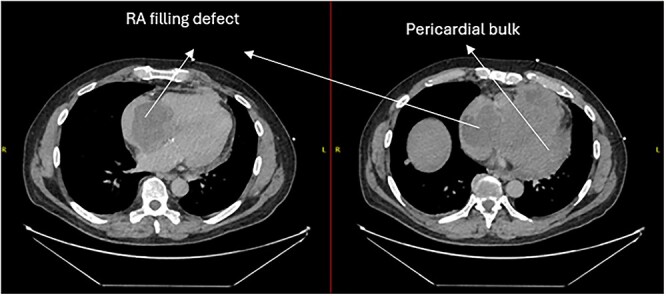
CT thorax-abdomen pelvis, depicting the RA filling defect as well as pericardial bulk.
